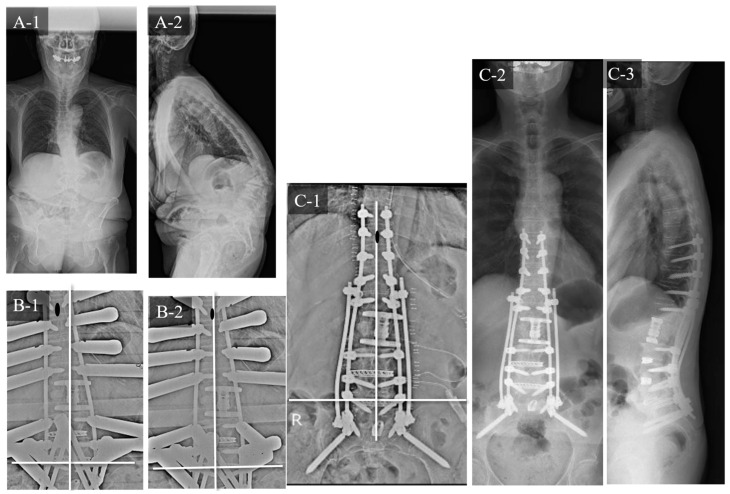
Case study 1 (RR). (A-1) Preoperative standing whole spine radiograph (AP). (A-2) Preoperative standing lateral whole spine radiograph. (B-1) Intraoperative radiograph before RR. (B-2) Intraoperative radiograph after RR. (C-1) Postoperative radiograph (AP). (C-2) Postoperative standing whole spine radiograph (AP). (C-3) Postoperative standing lateral whole spine radiograph. RR: rod rotation.
